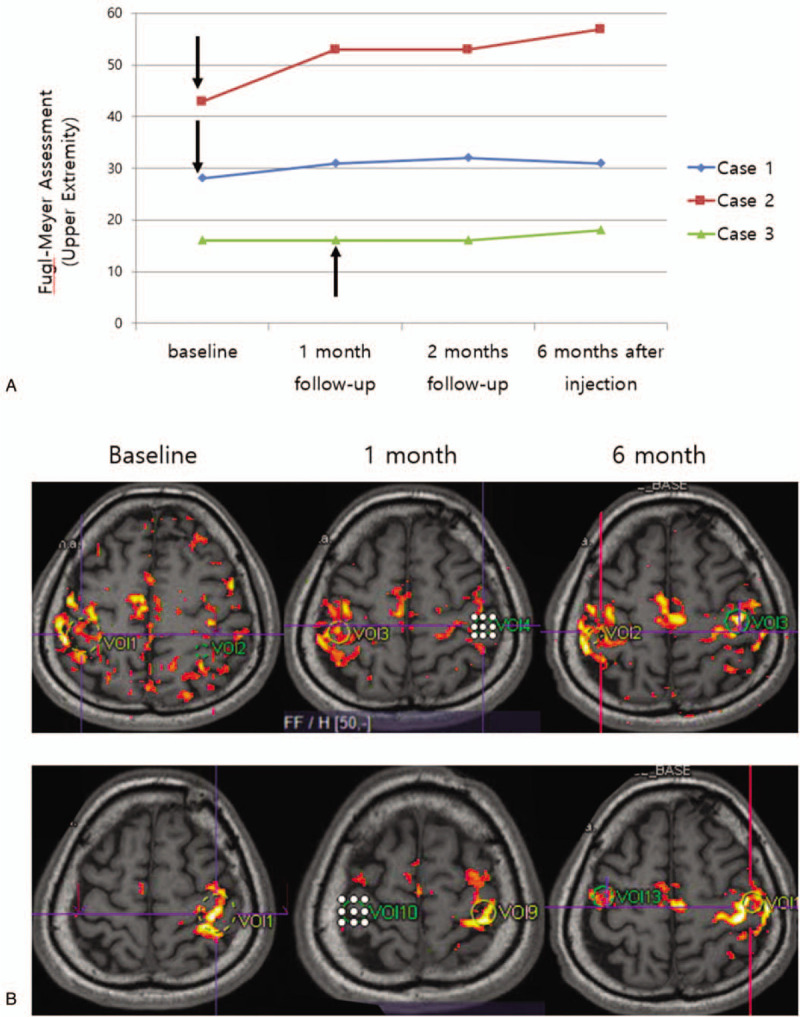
(A) Fugl–Meyer assessment for upper extremity of each cases before and after procedure. Arrows indicate time of injection. (B) Functional MRI changes over time in Case 2. Upper row is for moving the affected hand, and lower row is for moving the unaffected hand. See text for explanation. MRI = magnetic resonance imaging.
